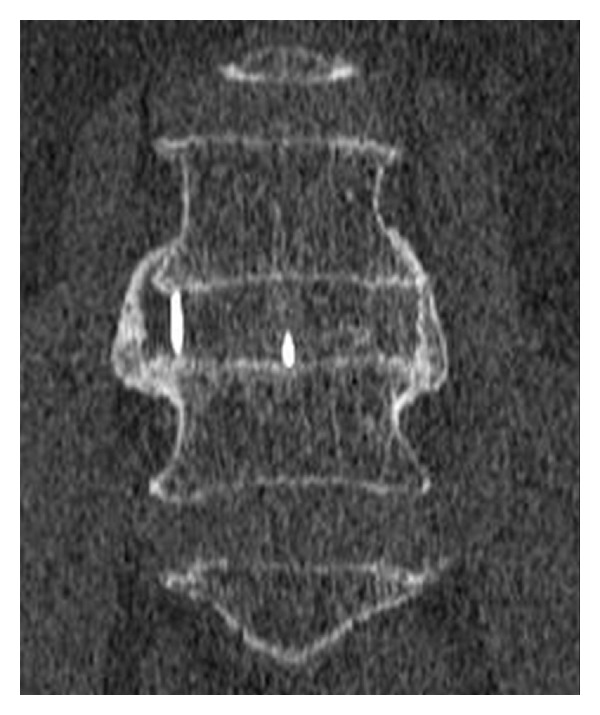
CT image demonstrating fusion.
